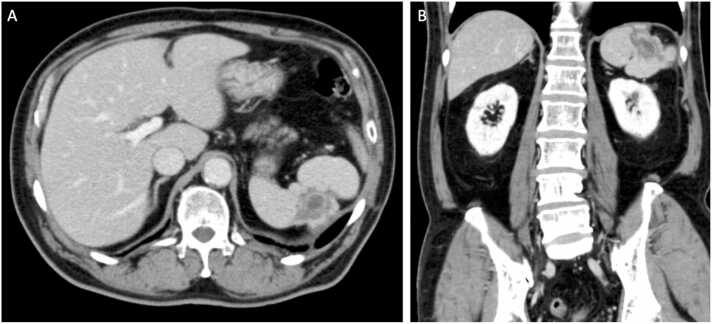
Follow-up CT scan performed 4 months after the discharge. Abscess cavity almost completely disappeared.
